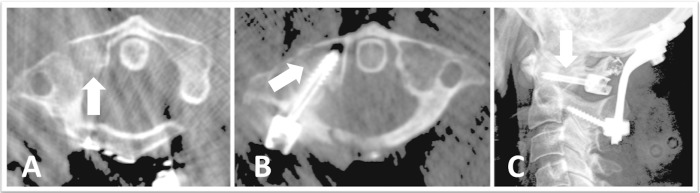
Intraoperative CT and postoperative x-ray after stage 1 An intraoperative axial computed tomography (CT) scan demonstrating the placement of a lag screw during the first stage and showing (A) the fracture line; (B) the lag screw passing through the lateral mass with fracture reduction; and (C) a postoperative x-ray demonstrating the occipitocervical instrumentation after the first stage of the procedure
